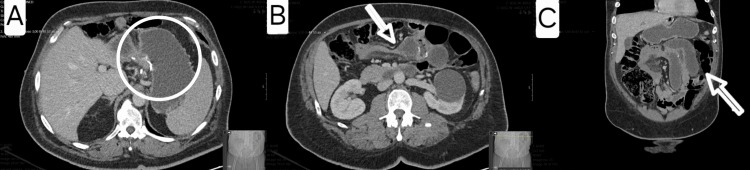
Case 5 - Triptych of abdominal CT images showing inconclusive but suggestive findings of internal hernia Sequential CT images from a contrast-enhanced abdominal scan: Image A (axial): The white circle highlights a congested mesenteric root with slight crowding of vessels. Image B (axial): The white arrow points to distended proximal small bowel loops. Image C (coronal): The white arrow indicates abrupt transition of caliber and clustered loops in the left upper quadrant. Despite the absence of a definitive swirl sign, these findings raised clinical suspicion for internal herniation. Surgical exploration confirmed mesenteric venous congestion without frank herniation, and Petersen’s space was closed prophylactically.
